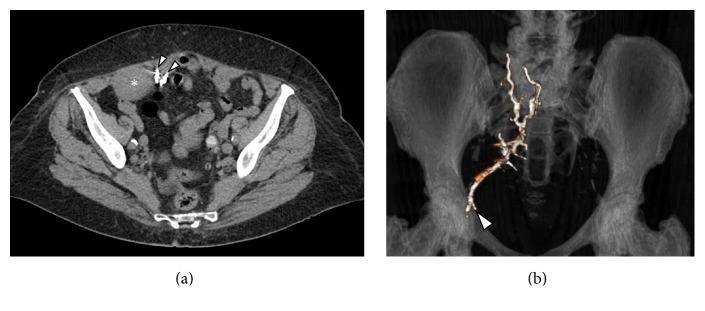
(a) MD-CT axial view at one month demonstrates the reduction of the haematoma into the right rectus sheath (*∗*) with two hyperdense artefact due to the Squidperi liquid embolic material. (b) MD-CT coronal Volume Rendering Technique image at three months with right epigastric artery reconstruction (arrowhead) filed by Squidperi 18 liquid embolic material.
